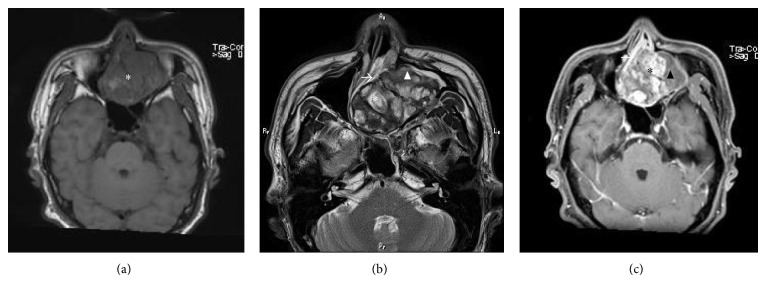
MRI findings of Case 1. (a) On T_1_-weighted imaging, the central region (∗) has a slightly lower intensity compared to that of the muscle. (b) On T_2_-weighted imaging, the central region (∗) demonstrates high intensity, and the periphery (▲) has lower intensity than the central region. Thickening of the paranasal mucosa is observed (→). (c) On contrast-enhanced T_1_-weighted imaging, the central region is strongly enhancing (∗), while the periphery (▲) demonstrates less enhancement than the central region. Additionally, the thickened mucosa of the paranasal sinus are strongly enhanced (→).
